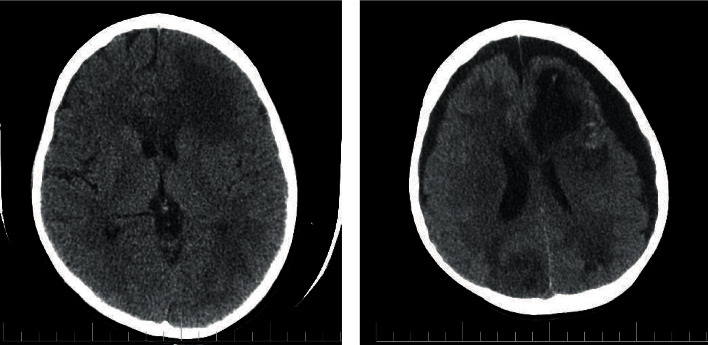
Brain CT scan: diffuse parenchymal hypodensity in left frontal and parietal lobes which suspected to a left-sided arterial stroke.
